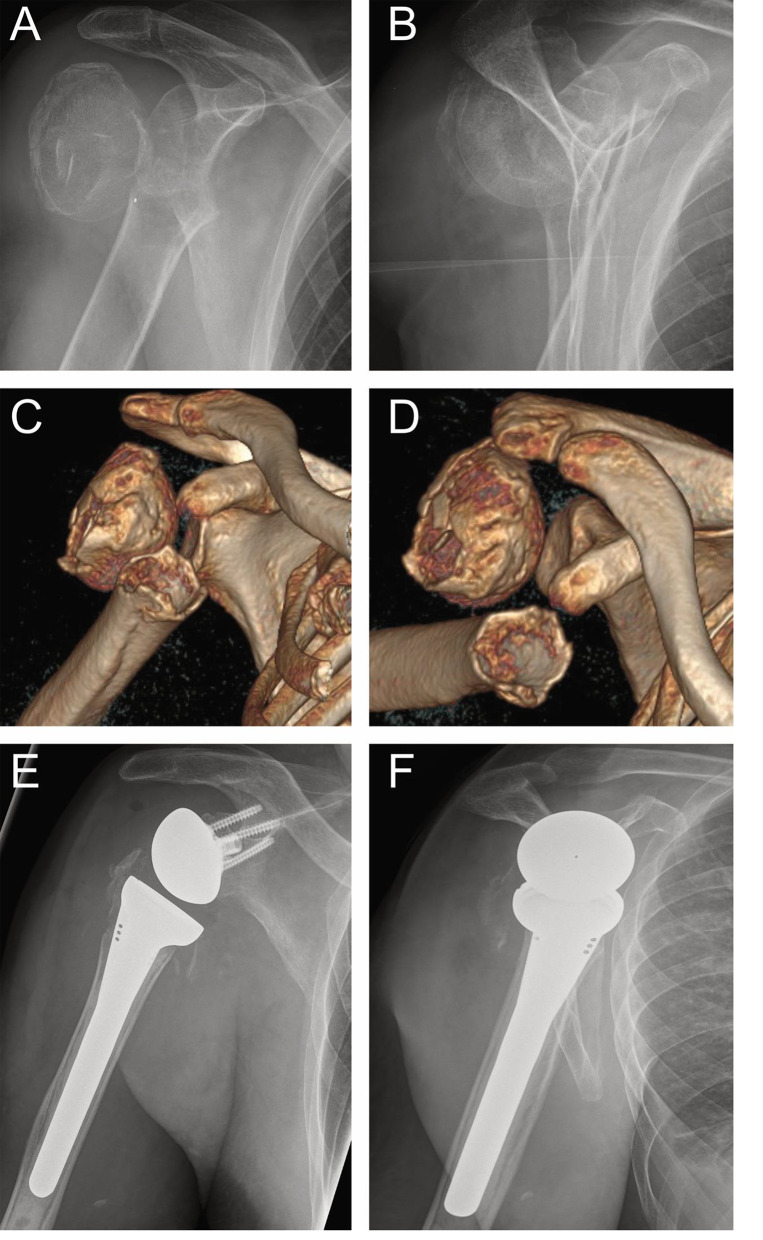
Preoperative a.p. (A) and y-view (B) X-ray of a complete head dislocation in an elderly patient, C, D 3D reconstruction of the fracture with head split component, and after surgical treatment with inverse shoulder endoprosthesis as a.p. X-ray (E) and y-view X-ray (F)
